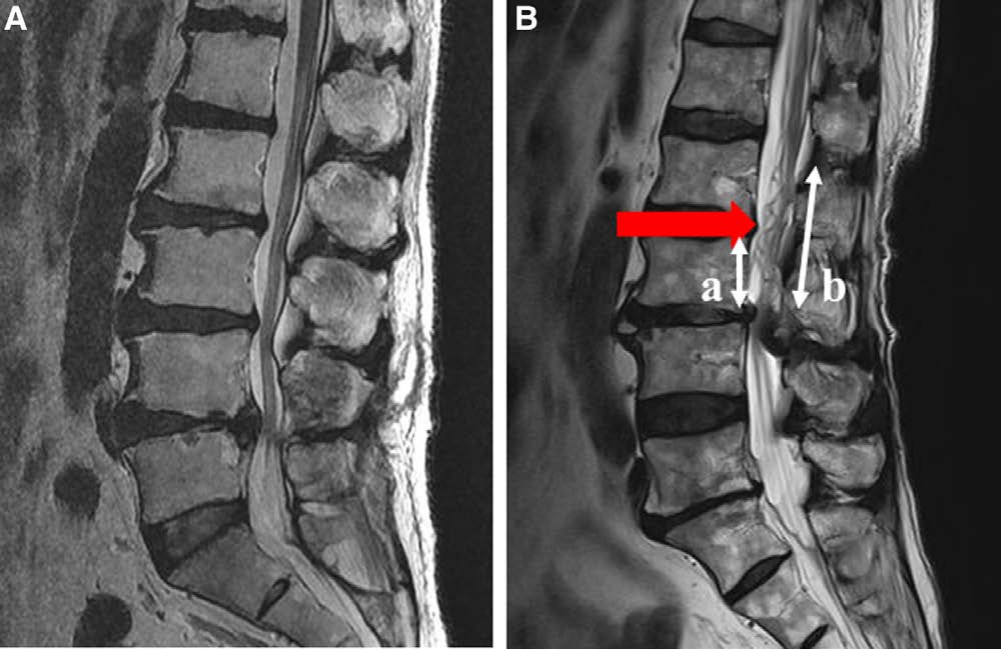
(A) Sagittal T2-weighted MR image showing normal distribution of the nerve roots. (B) Sagittal T2-weighted MR image showing a Serpentine pattern in the cauda equina (single-headed red arrow). The relative length of the “Serpentine pattern” was calculated by dividing the “Serpentine pattern” length (b) by the height of the body at the level of the stenosis (a).
